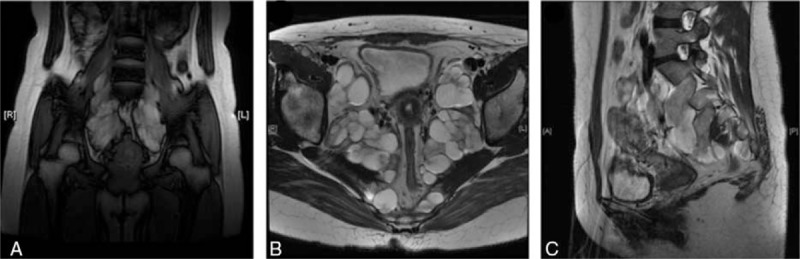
MRI demonstrated enlargement of lumbosacral nerves and abnormal signal on T2-weighted images in (A) the coronal, (B) the horizontal, (C) and the sagittal planes.
